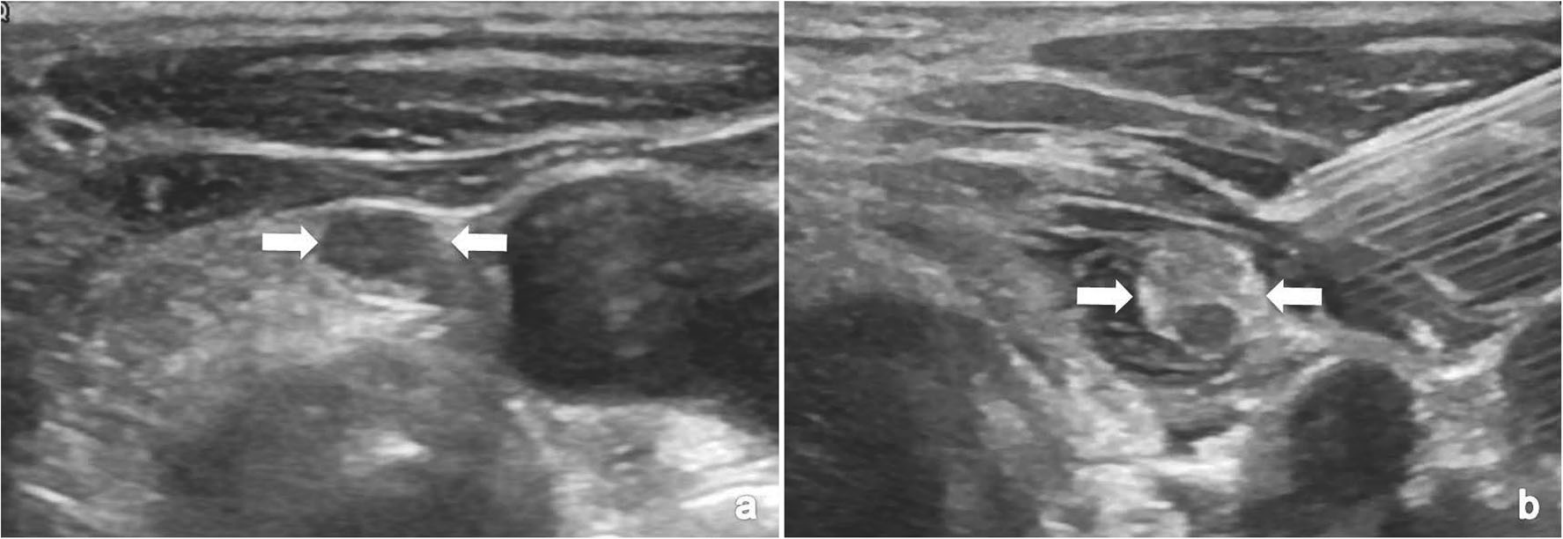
The hypoechoic hyperplastic parathyroid nodule and hyperechoic normal parathyroid tissue could be clearly visualized after establishment of hydrodissection. a Routine US showed a hypoechoic pHPT nodule (white arrows) in a 45-year female patients. b After the injection of isolation solution, the hypoechoic hyperplasic parathyroid nodule (white arrows) and hyperechoic normal parathyroid tissue were clearly displayed on US. pHPT, primary hyperparathyroidism; US, ultrasound
